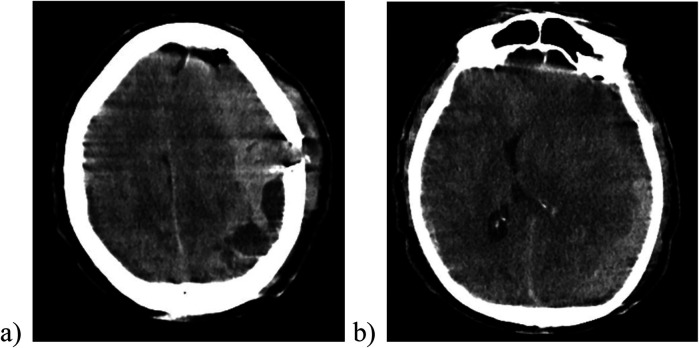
The cranial CT scan after removal of the subgaleal drainage revealing a massive sudden re-bleeding on the left side with midline shift to the right (image a), compression of the brain parenchyma and the lateral ventricle (image b).
